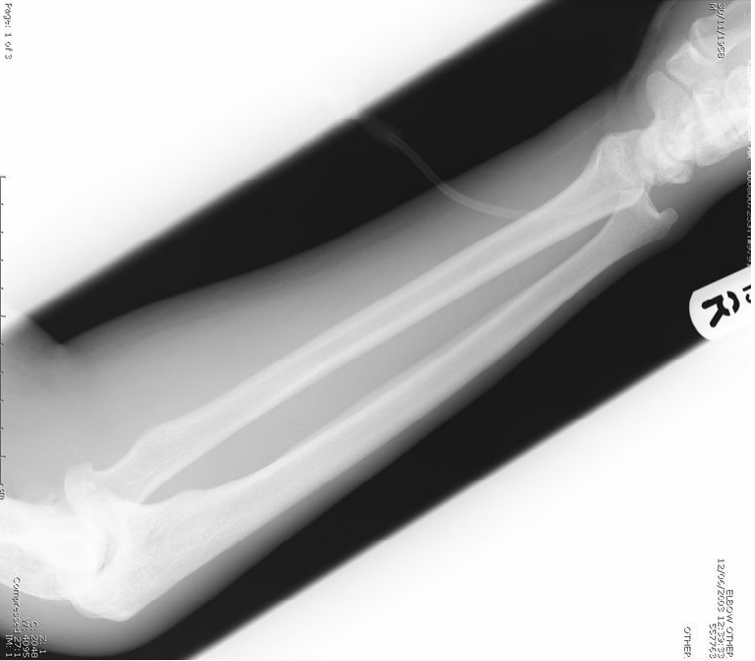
Radiograph of the elbow showing a dislocated radial head.
